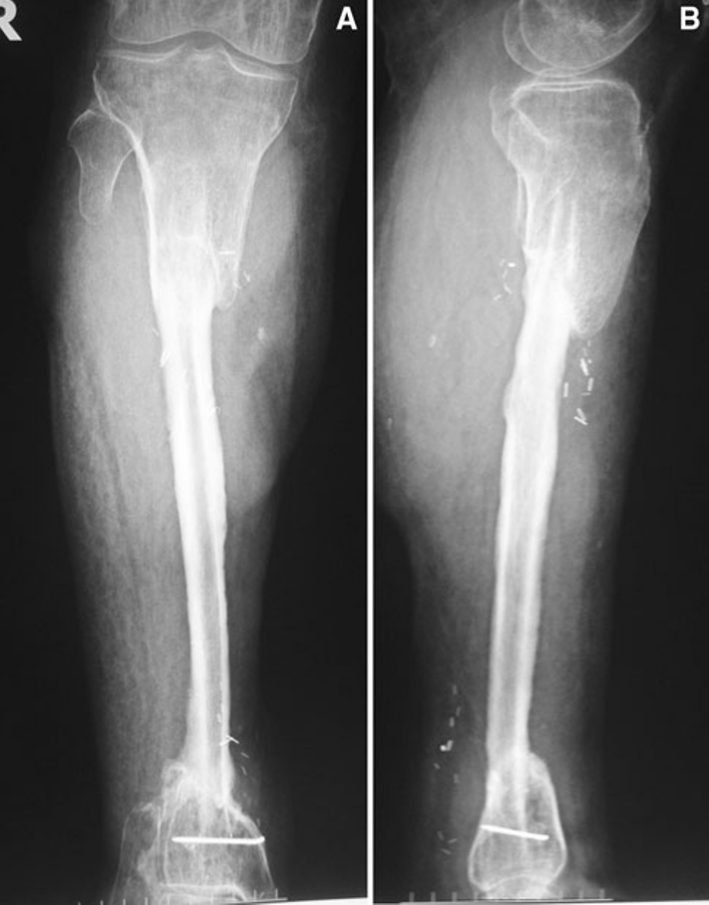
a, b Radiographs obtained 2 years after surgery show that the transferred fibula has been fully consolidated to allow full weight bearing. Thickening is well demonstrated
